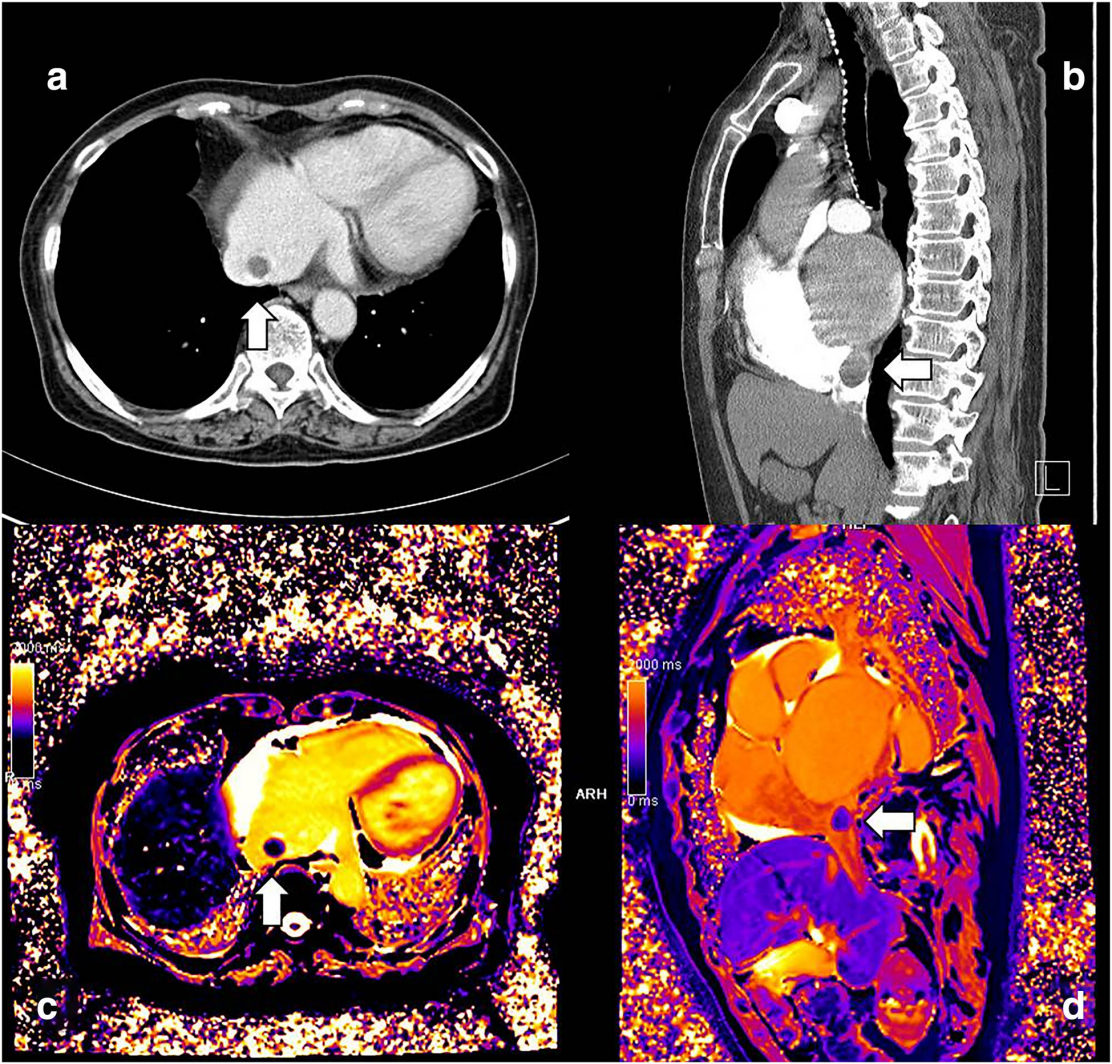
Preoperative computed tomography (a, b) and magnetic resonance (c, d) studies disclosed a smooth, rounded mass within right atrium (arrows)
